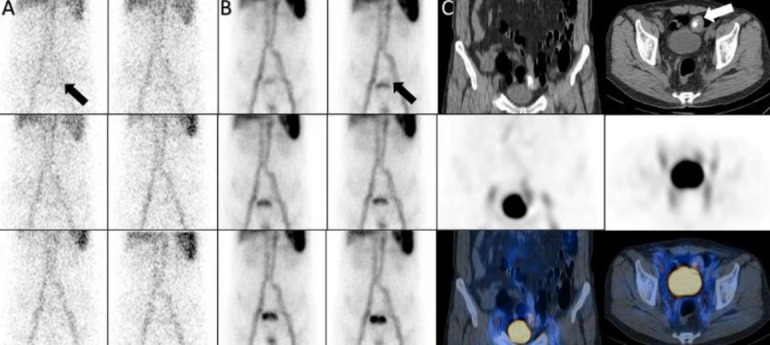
Perfusion and dynamic images showed the suspicious area of increased activity adjacent to the bladder (arrows in A, B). SPECT/CT images showed the source of bleeding was benign vascular lesion in the ileum (arrow, C)
